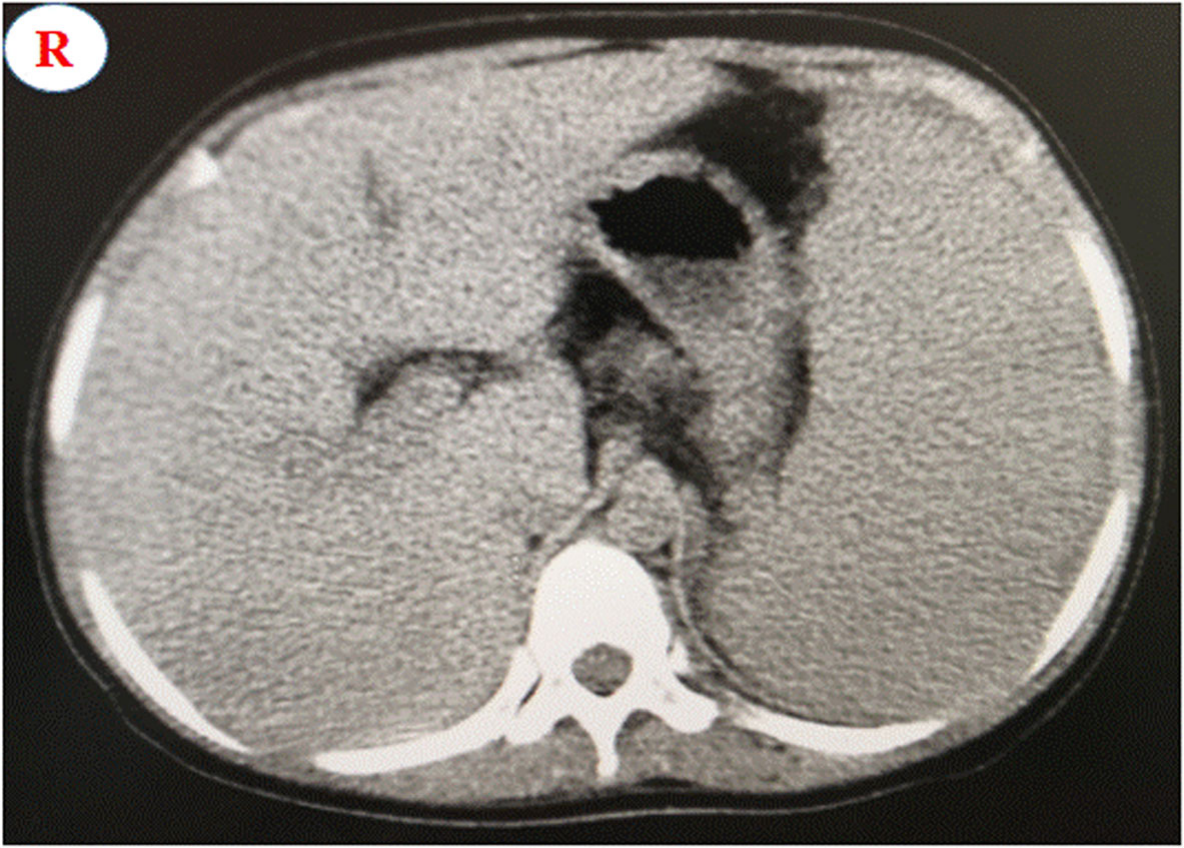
Abdominal contrast-enhanced CT revealed splenomegaly
